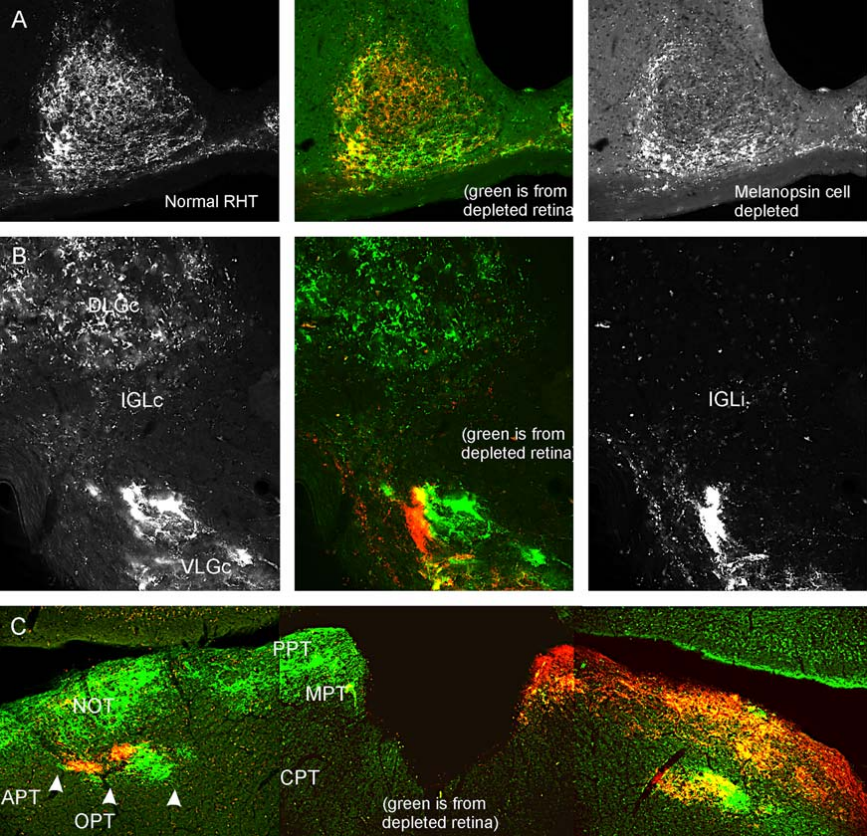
UF008/SAP greatly reduces retinal projections to the suprachiasmatic nucleus and intergeniculate leaflet. CT-B tracing of RHT projections remaining after intravitreal UF008/SAP injection shows a terminal field densest along the lateral, ventrolateral and ventral SCN border, but greatly reduced in, or absent from, the dorso-central SCN. B) In the IGL, the contralateral projection from the UF008/SAP-injected retina is largely absent. C) There are no readily apparent differences with respect to the remaining retinal innervation of the OPT. The red label in all parts of the figure identifies terminals from the contralateral, undamaged retina. Abbreviations: APT – anterior pretectal n.; CPT – commissural pretectal n.; DLGc – dorsal lateral geniculate n;, contralateral; IGLc – intergeniculate leaflet, contralateral; IGLi – intergeniculate leaflet, ipsilateral; MPT – medial pretectal n.; NOT – nucleus of the optic tract; PPT – posterior pretectal n.; RHT – retinohypothalamic tract; VLGc – ventral lateral geniculate n., contralateral. (Ipsi- and contra- are referenced with respect to the injected with UF008/SAP).
